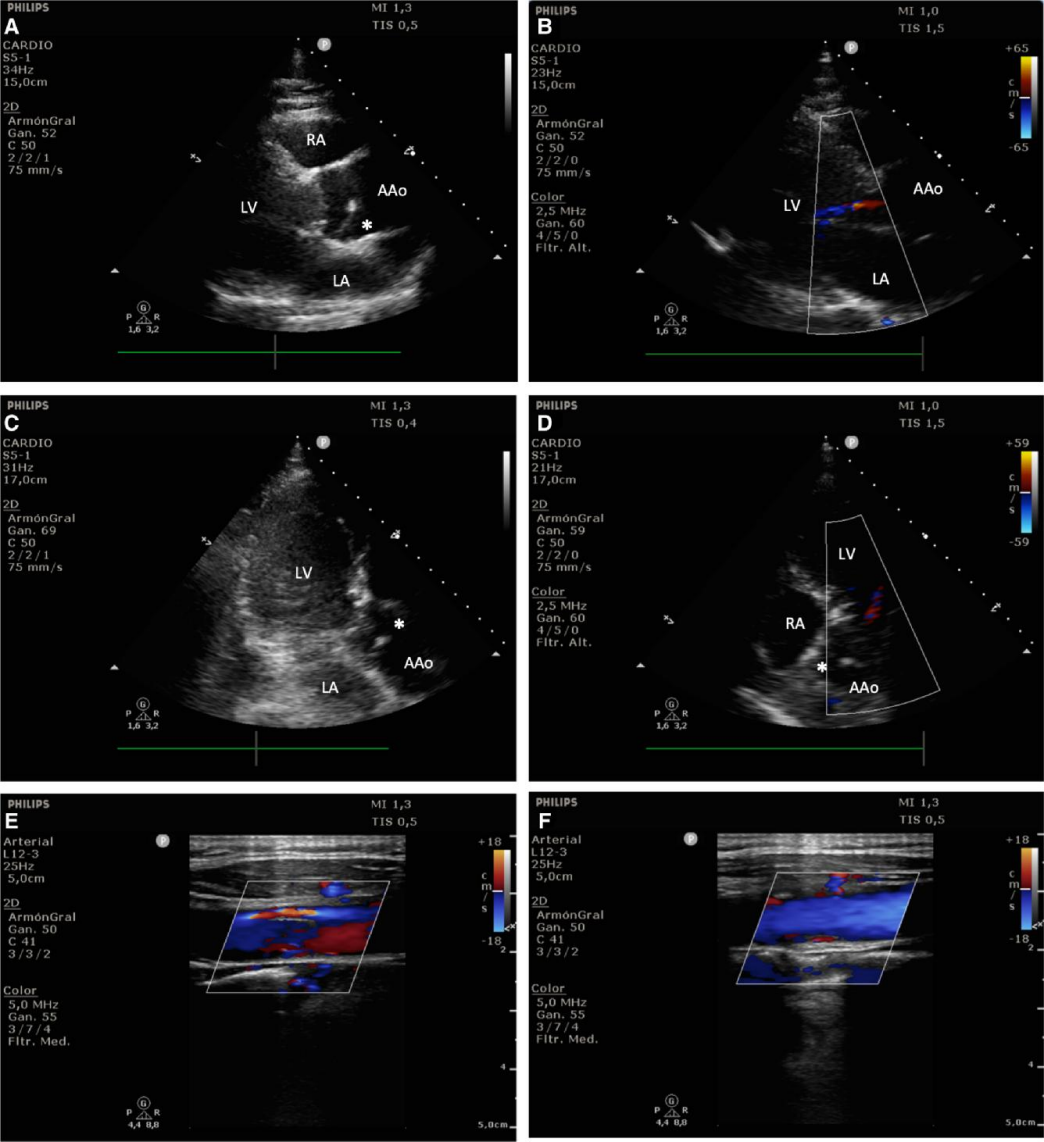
Point-of-care ultrasound images taken at admission. (*A*) Cardiac ultrasound parasternal long-axis plane showing the image of an intimal flap in the ascending aorta (*). (*B*) Doppler parasternal long axis showing a mild-to-moderate aortic insufficiency. (*C*) Apical three-chamber view showing the intimal image flap (*). (*D*) Apical five-chamber view where the intimal flap and aortic insufficiency are present. (*E*) Left common carotid artery with a false lumen and turbulent blood flow through the smaller true lumen. (*F*) Normal right common carotid artery. AAo, ascending aorta; LA, left atrium; LV, left ventricle; RA, right atrium.
